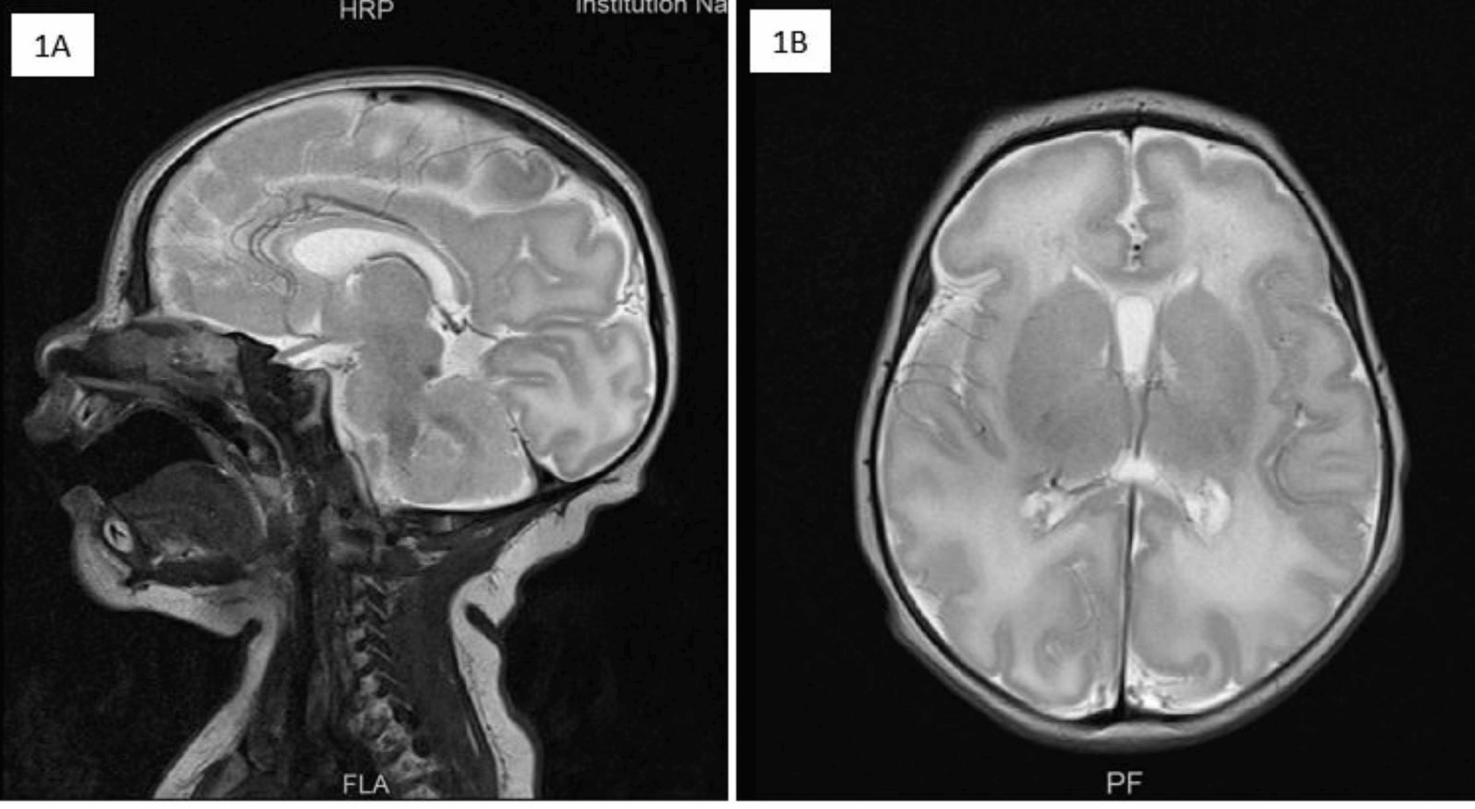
(A) Mid sagittal T2 weighted scan shows presence of normal brain parenchyma without any evidence of atrophy; (B) Axial T2-weighted scan at the level of lateral ventricles and deep grey nuclei shows normal bilateral supratentorial brain parenchyma
